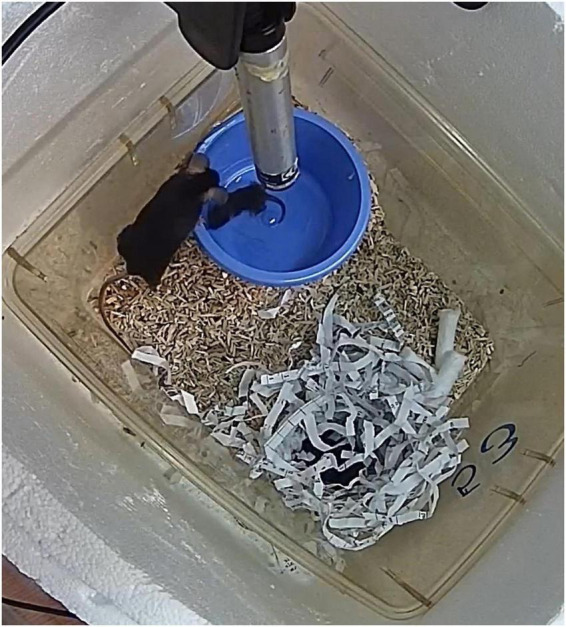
Image of the BAMBI testing setup. BAMBI test was performed in the home-cage and included a cup on PND7-13 to prevent the pups from crawling back into the nest. An ultrasound microphone was placed approximately 5 cm above the test pup’s corner in order to minimize interference from USVs emitted by the pups in the nest (in the opposite corner). A video camera was mounted above the home-cage.
